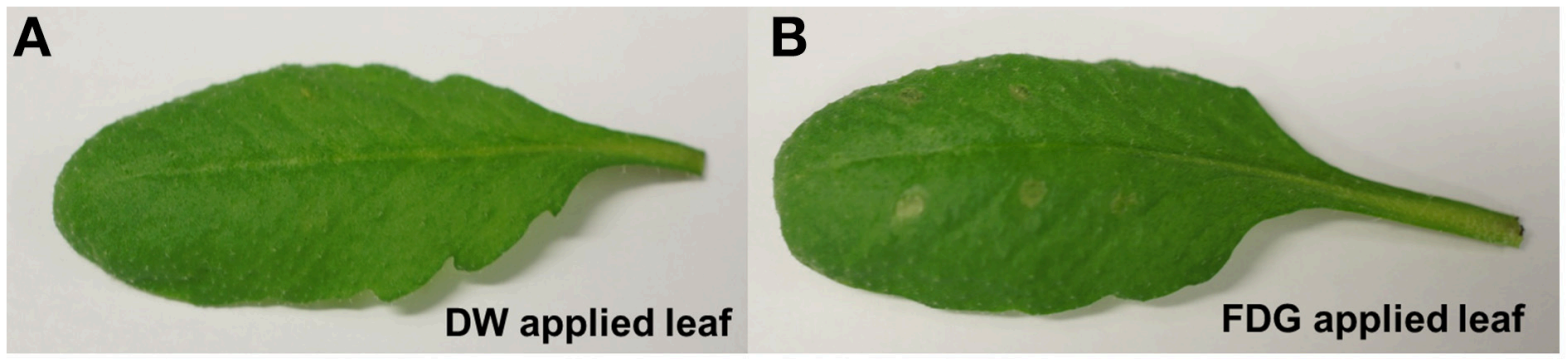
FDG application affected local leaf tissue. Mature leaf was scratched on abaxial surface at 6 spots and 5 μL of distilled water (DW) (A), FDG (20 mg.mL^−1^) (B) was applied locally on each spot. Leaf pictures were taken after 4 h. Dehydrated spots were visible at FDG application site.
